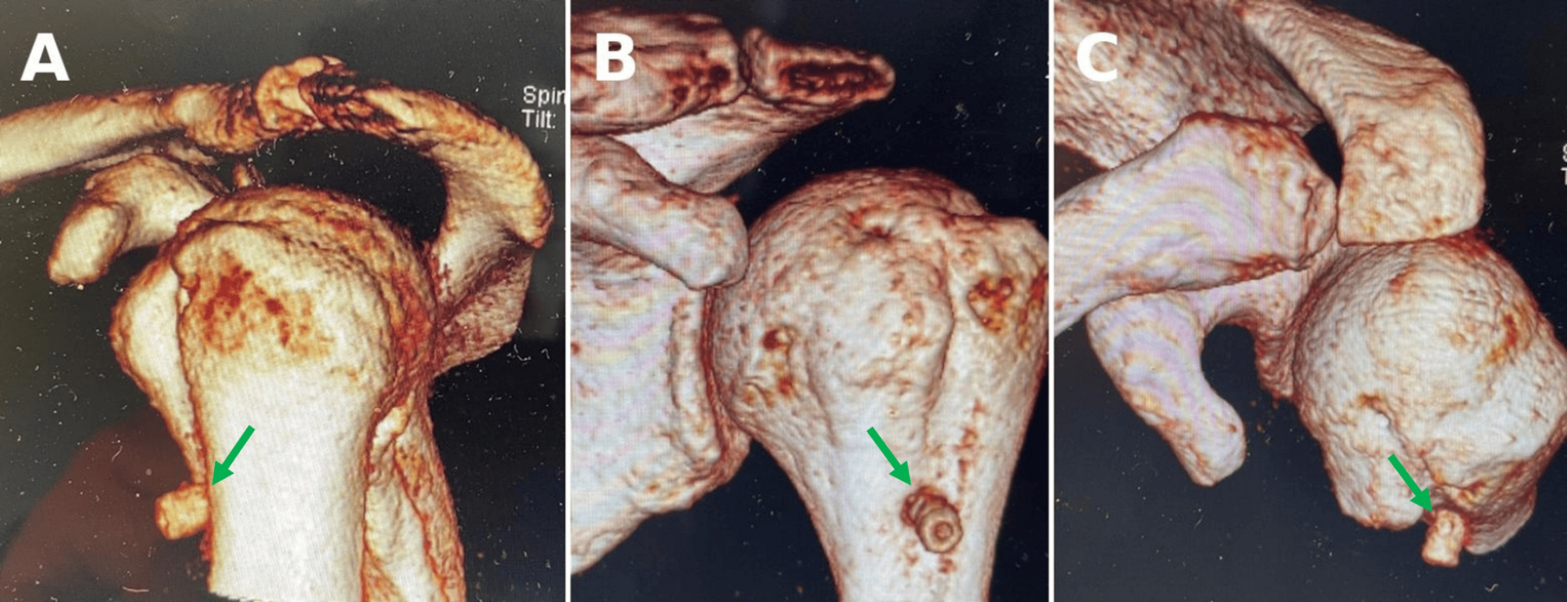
Eight weeks postoperative CT of the left shoulder (three-dimensional reconstruction) demonstrating cut-out screw position in different views. (A-C) Visible screw penetration (green arrows). CT: computed tomography.
